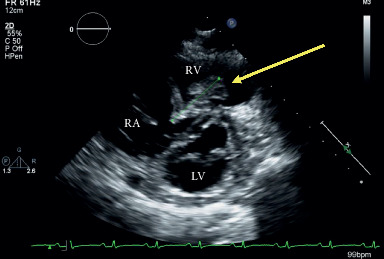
Parasternal short-axis view at the mitral valve level, demonstrating a dilated right ventricle, a D-shaped interventricular septum, and a large multilobulated mass in the right ventricle (arrow). RA: right atrium; RV: right ventricle; LV: left ventricle.
